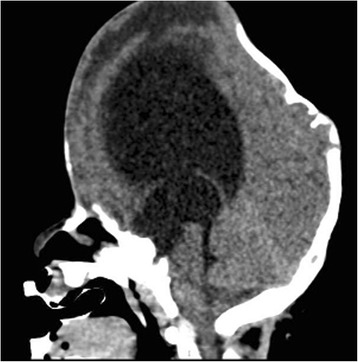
Sagittal MR image shows marked ventricular dilatation in our patient affected by Pfeiffer syndrome
